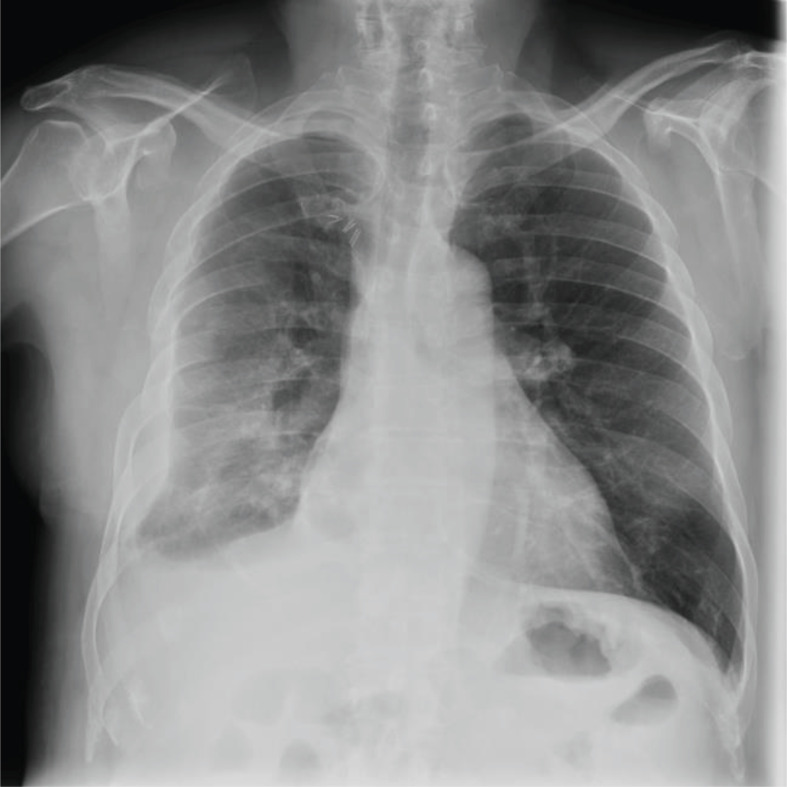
Chest X-ray after resection (1 month).
